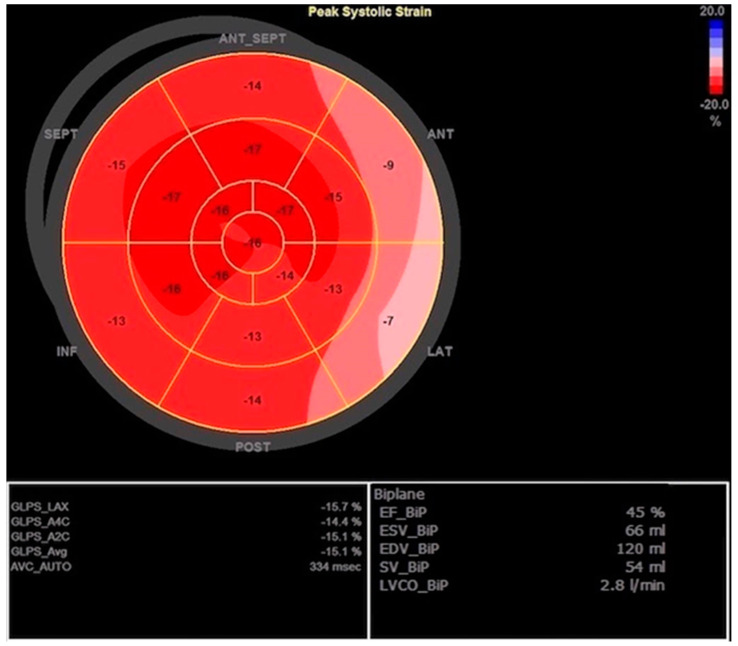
Initial transthoracic echocardiography with mildly reduced left ventricular ejection fraction (EF) calculated using Simpson biplane method and reduced global longitudinal peak strain (GLPS) with reduced values in basal segment of the anterior, septal, and lateral walls.
